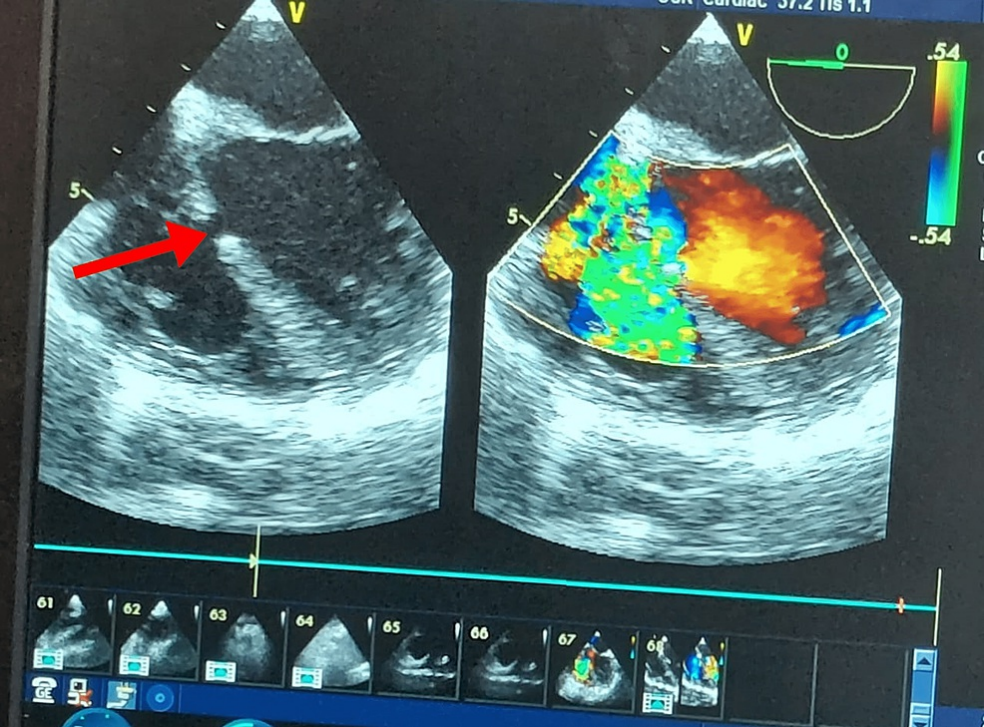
2D echocardiography monitor image showing large ventricular septal defect.
